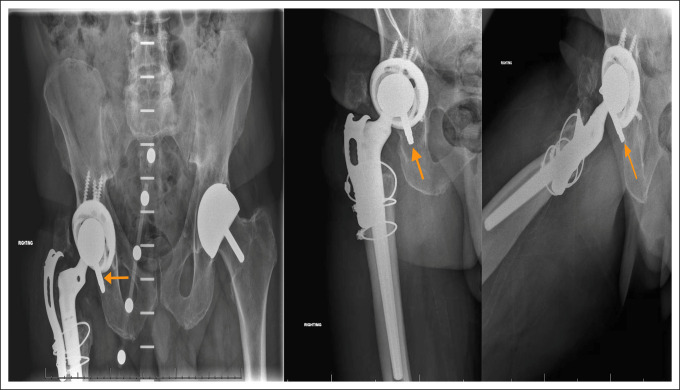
Radiographs from the 20-month follow-up on January 27, 2023.
